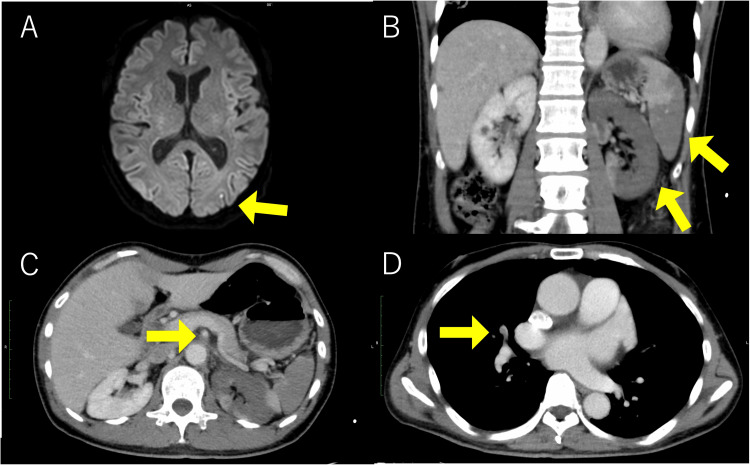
Contrast-enhanced CT and MRI findings (A) Diffusion-weighted MRI showing high signal intensity consistent with acute cerebral infarction in the left occipital lobe. (B) Contrast-enhanced CT demonstrating infarctions in the kidneys and spleen. (C) A superior mesenteric artery (SMA) thrombus is identified. (D) A contrast defect is observed in the right pulmonary artery, indicative of pulmonary embolism CT: computed tomography; MRI: magnetic resonance imaging
